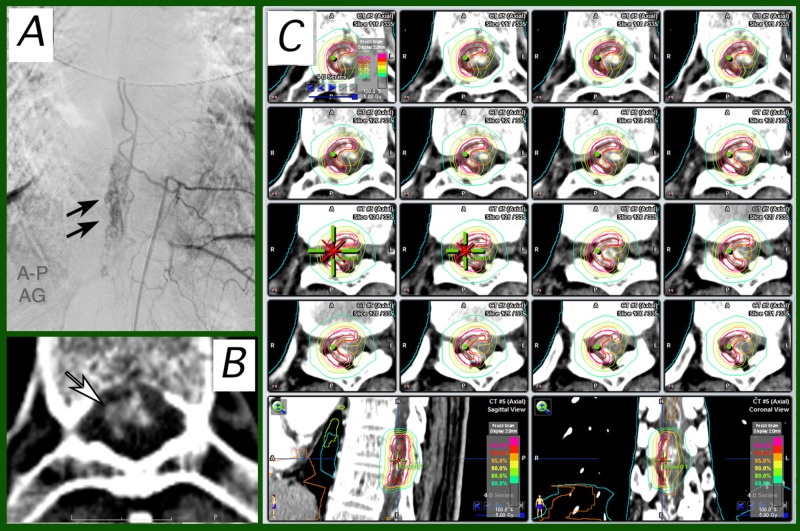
Case 5 Frontal view of left spinal angiography (5A) and axial CT (5B) before stereotactic radiotherapy (SRT). A little longitudinally long and narrow nidus was demonstrated (arrows, 5A). The nidus and draining veins were revealed as an irregular-shaped contrast-enhancement area in the spinal canal (white arrow, 5B) on CT. As a provocation test worsened the neurological signs, embolization was not performed. 5C: dose-planning of SRT on the iPlan workstation. Seven-beam intensity-modulated SRT plan was employed.
